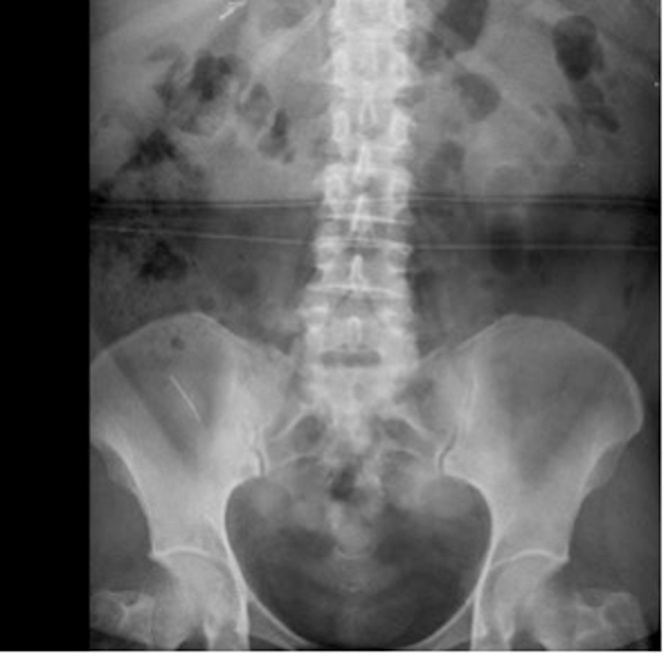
Radiograph showing metal density foreign body in the lower right quadrant.
